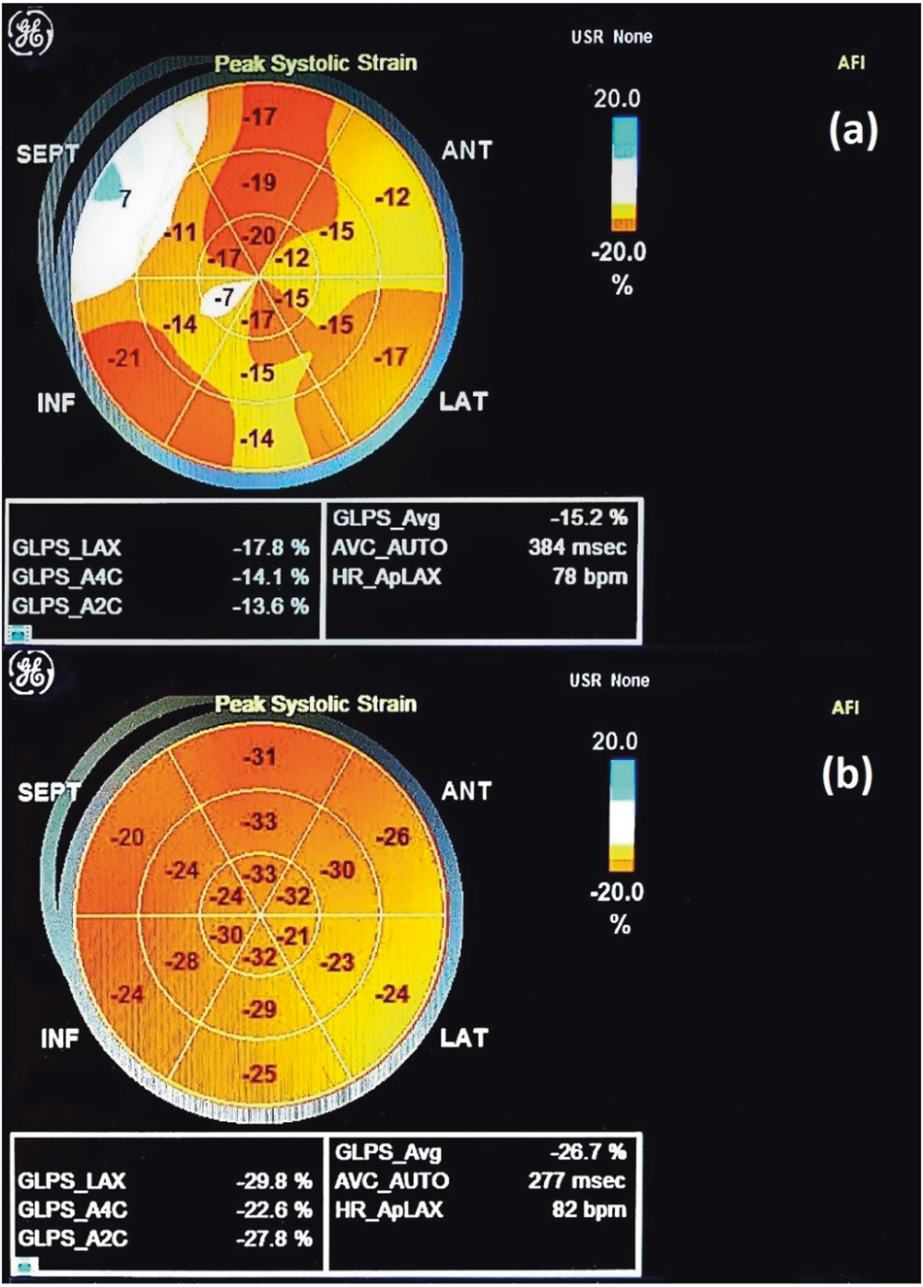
Peak systolic strain and global longitudinal strain assessment using speckle tracking echocardiography. a Abnormal values and b normal values.
